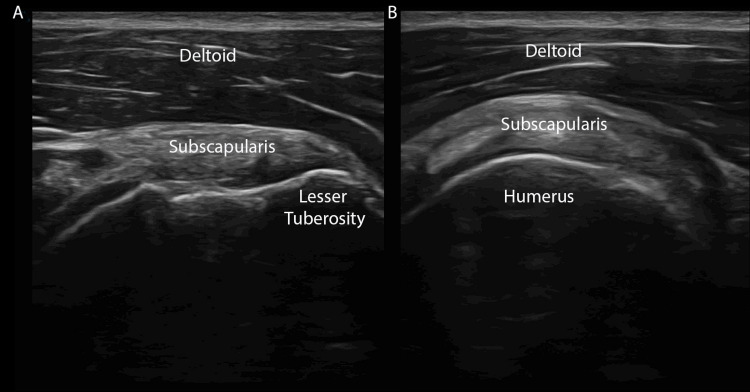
Subscapularis (A) Long axis view of the subscapular tendon. The bird’s beak appearance as it attaches to the lesser tuberosity is demonstrated. (B) Rotation of the ultrasound probe 90 degrees clockwise will yield a transverse view of the subscapularis and the subscapularis’ myotendinous structure with characteristic fibular tendinous anisotropic bundles.
